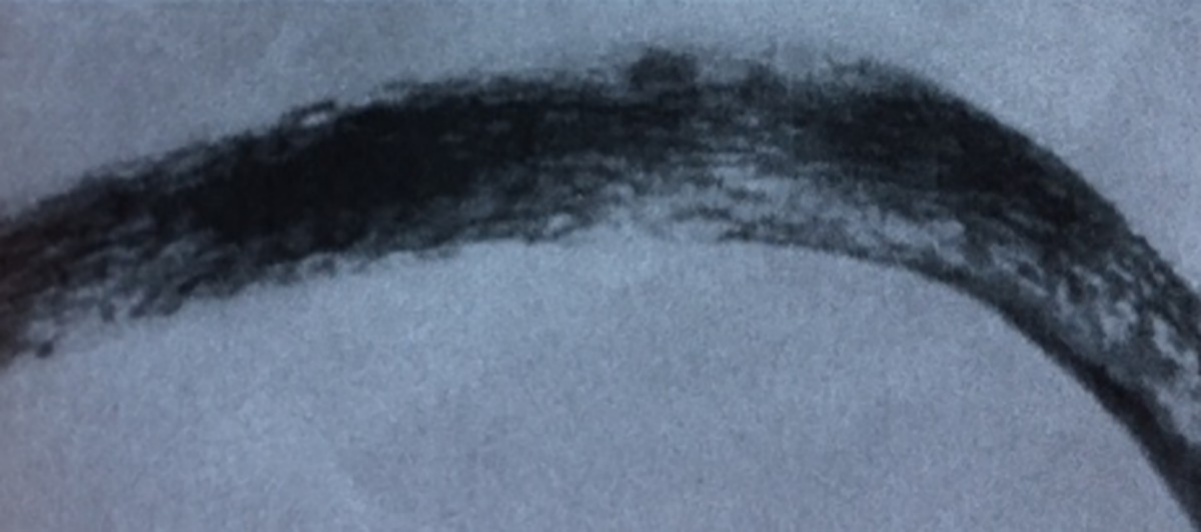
Radiograph of an artificially decalcified rib, with 54.7% of the calcium removed. From: Lachman E and Whelan M.A: The roentgen diagnosis of osteoporosis and its limitations. Radiology 26, 165–177 (1936) (with permission).
